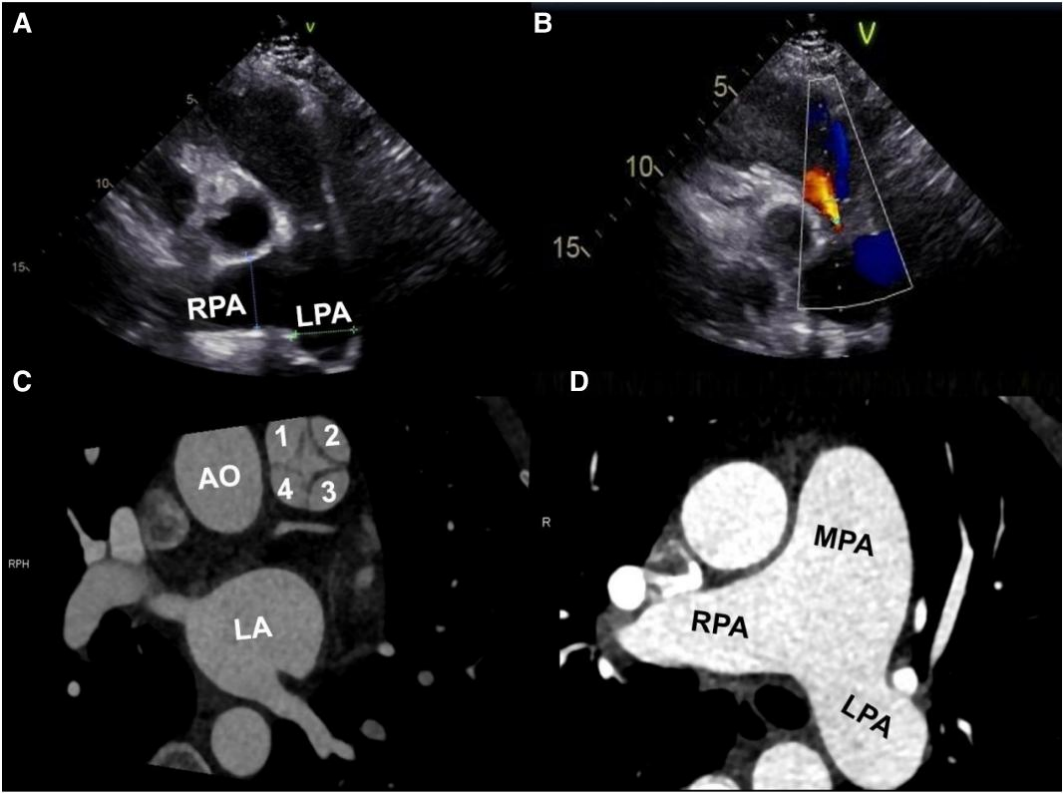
Images in a 52-year-old woman. (*A*) The transthoracic echocardiographic image revealed dilation of the pulmonary arteries of the left (25 mm) and right (28 mm). (*B*) Colour Doppler imaging showed mild pulmonary valve regurgitation and systolic flow acceleration time in the right ventricular outflow tract (63 ms). (*C* and *D*) The computed tomography angiography scan demonstrated a quadricuspid pulmonary valve (leaflets are numbered 1, 2, 3, and 4) with one smaller cusp (leaflet number 4) and a dilated main pulmonary artery (33 mm). AO, aorta; LA, left atrium; MPA, main pulmonary artery; RPA, right pulmonary artery; LPA, left pulmonary artery.
